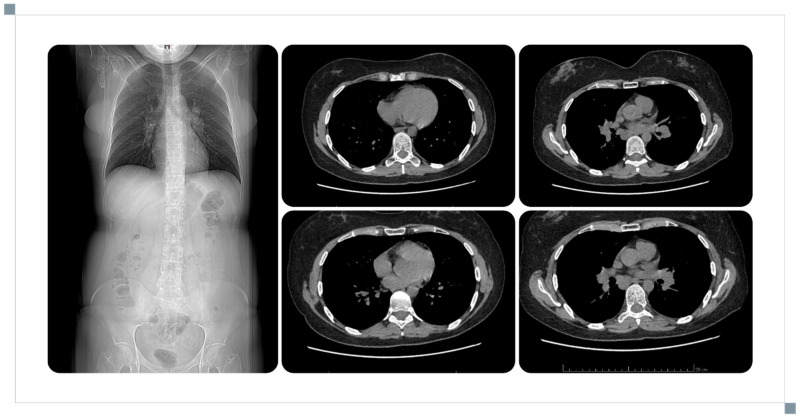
Chest CT scan with lesions typical of sarcoidosis.
